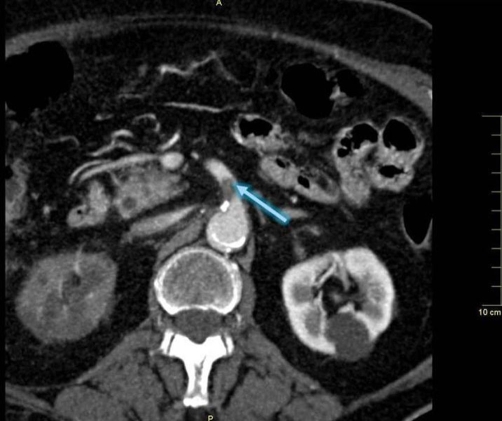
CT scan image showing thrombosis of the proximal portion of the superior mesenteric artery (arrow). A lack of contrast enhancement of the right kidney can also be appreciated.
